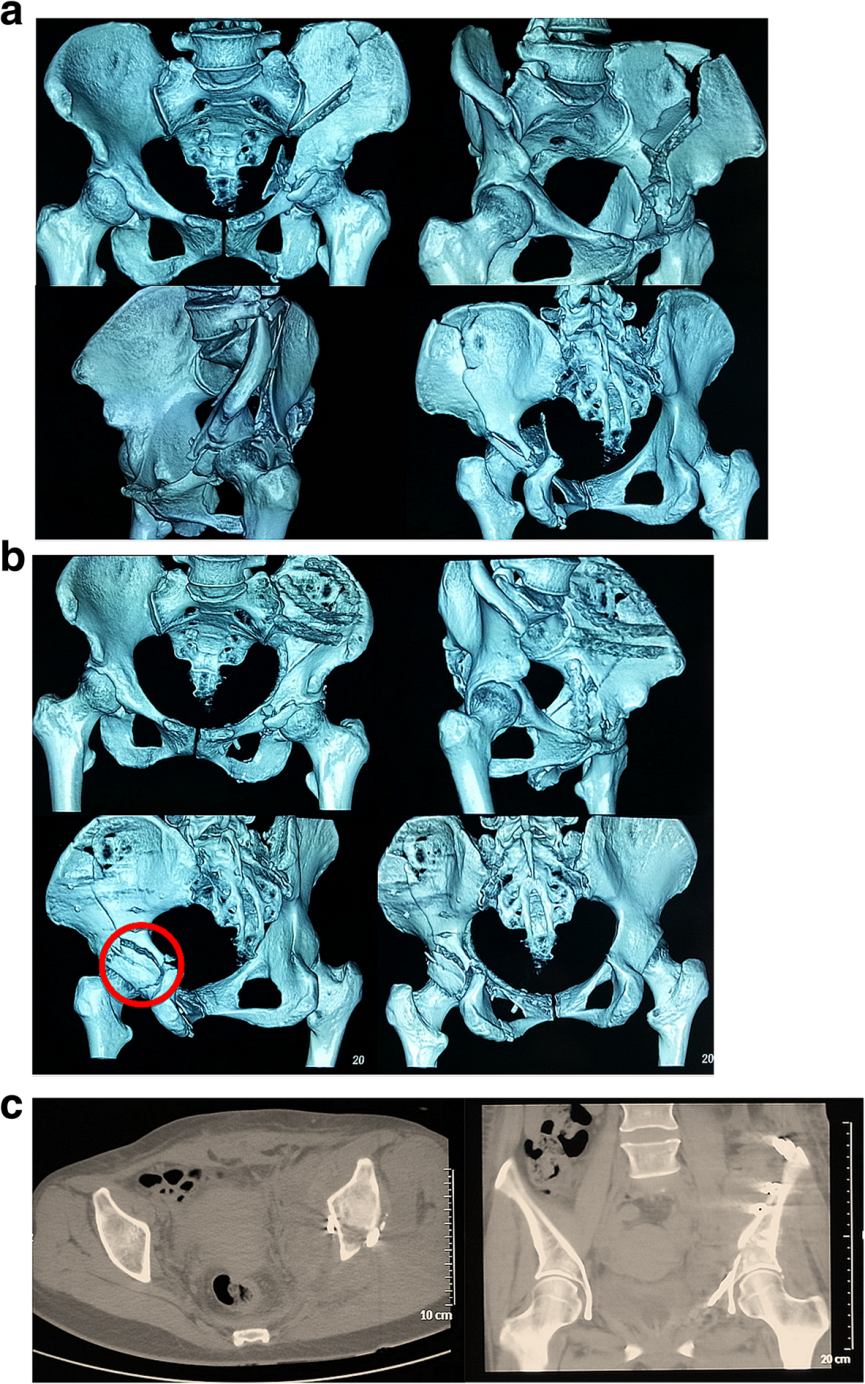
Images for a 45-year-old patient due to a traffic accident. a Preoperative computed tomography scans showed a both-column acetabular fracture including high anterior column fracture (fracture line B in the study) and posterior column fracture (fracture line C in the study). b high anterior column fracture (fracture line B in the study) was treated through the Stoppa and iliac fossa approach and sequential Kocher-Langenbeck approach was added because of the displaced posterior-wall fragment (red circle). c Postoperative coronal and axial CT scans at the level of the acetabular dome presenting anatomic reduction
